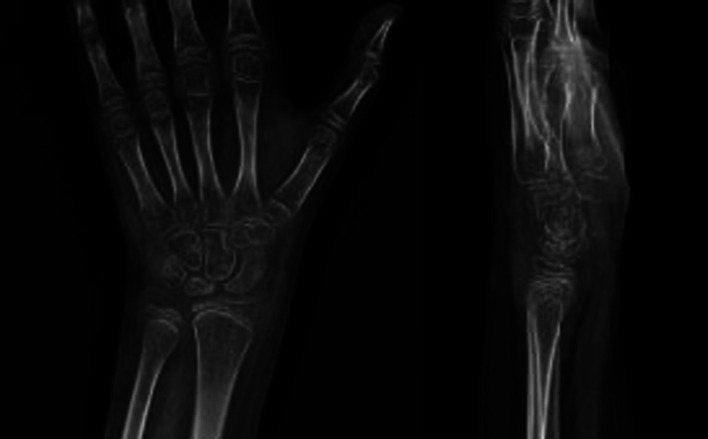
An injured wrist X-ray 10 months after the surgery.
